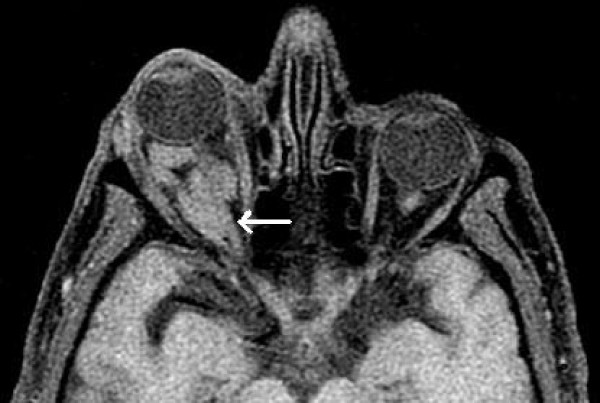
Axial MR image (T1 with fat saturation). An isointense to brain and optic nerve (arrow) lesion which produces exopthalmos. The lesion appears as marked widening along the path of the optic nerve but there is no intracranial extension.
